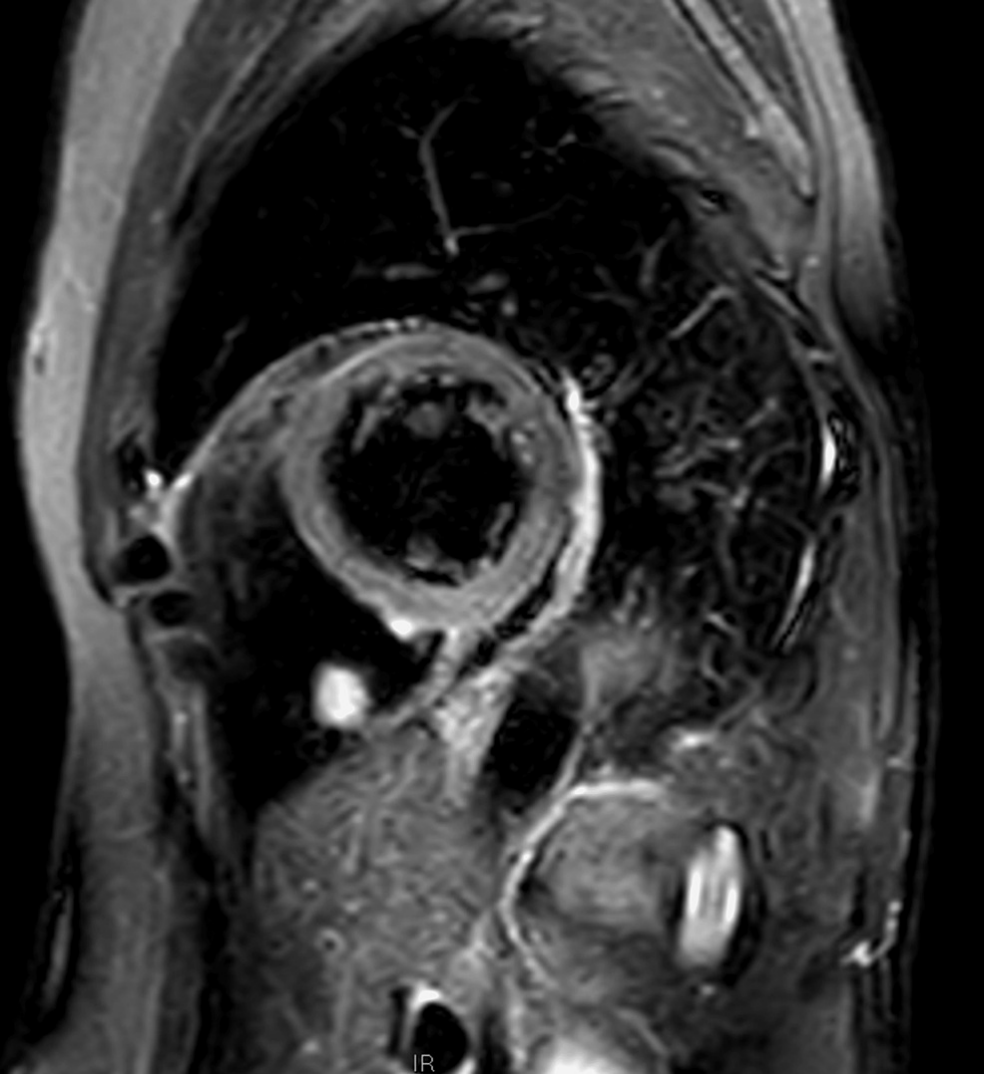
T2-weighted scan of the right ventricular mass
